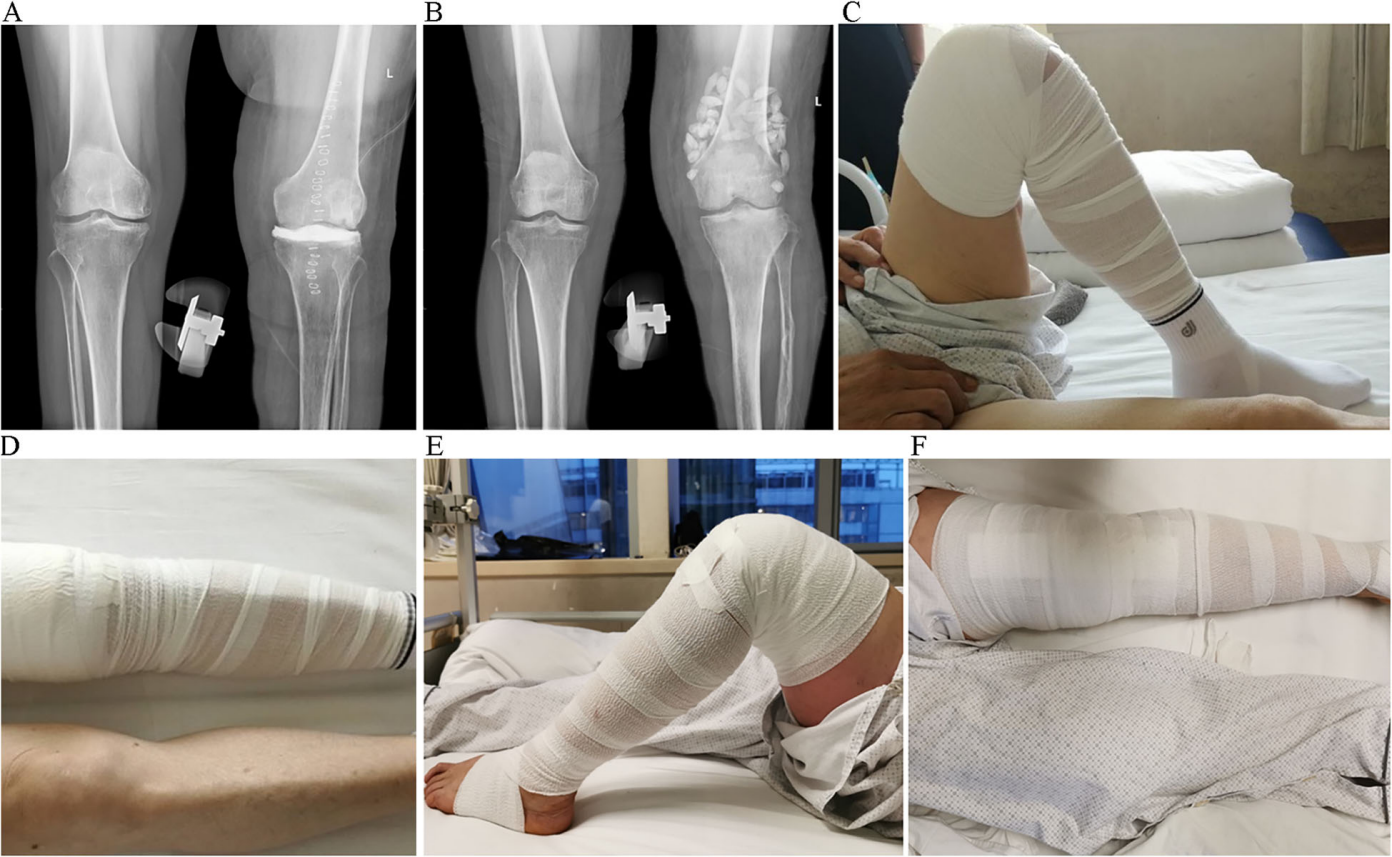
a X-ray of the knee joint after tibial plateau spacer implantation. b X-ray of the knee joint after antibiotic cement bead implantation. c, d Functional photos of a patient with tibial plateau spacer, whose ROM was improved from 10–100° preoperative to 0–110° during the interval period. e, f Functional photos of a patient with antibiotic cement beads, whose ROM was improved from 10–80° preoperative to 0–90° during the interval period
